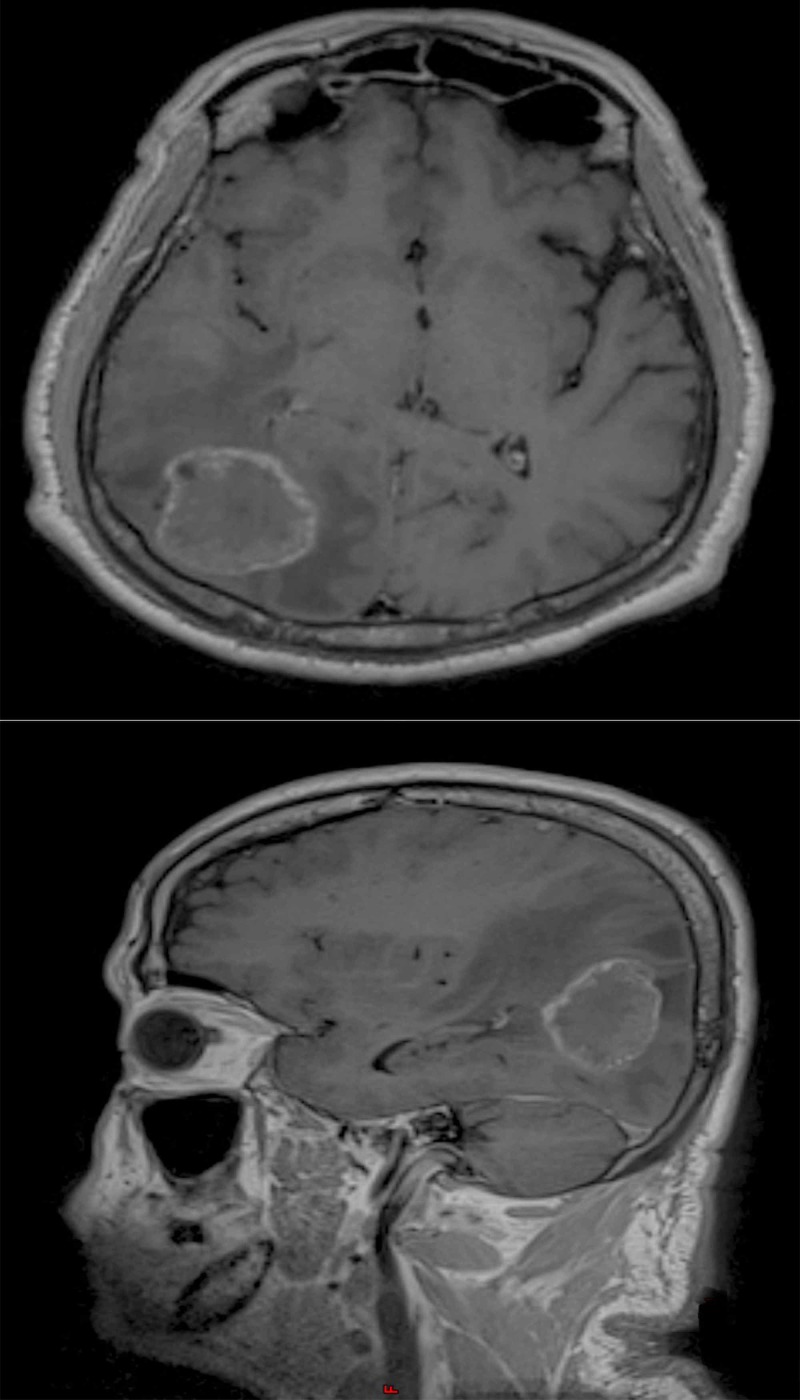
Preoperative MRI T1 sequence with contrast showing axial view (upper) and sagittal view (lower) of a space-occupying lesion in the occipital-parietal lobe
